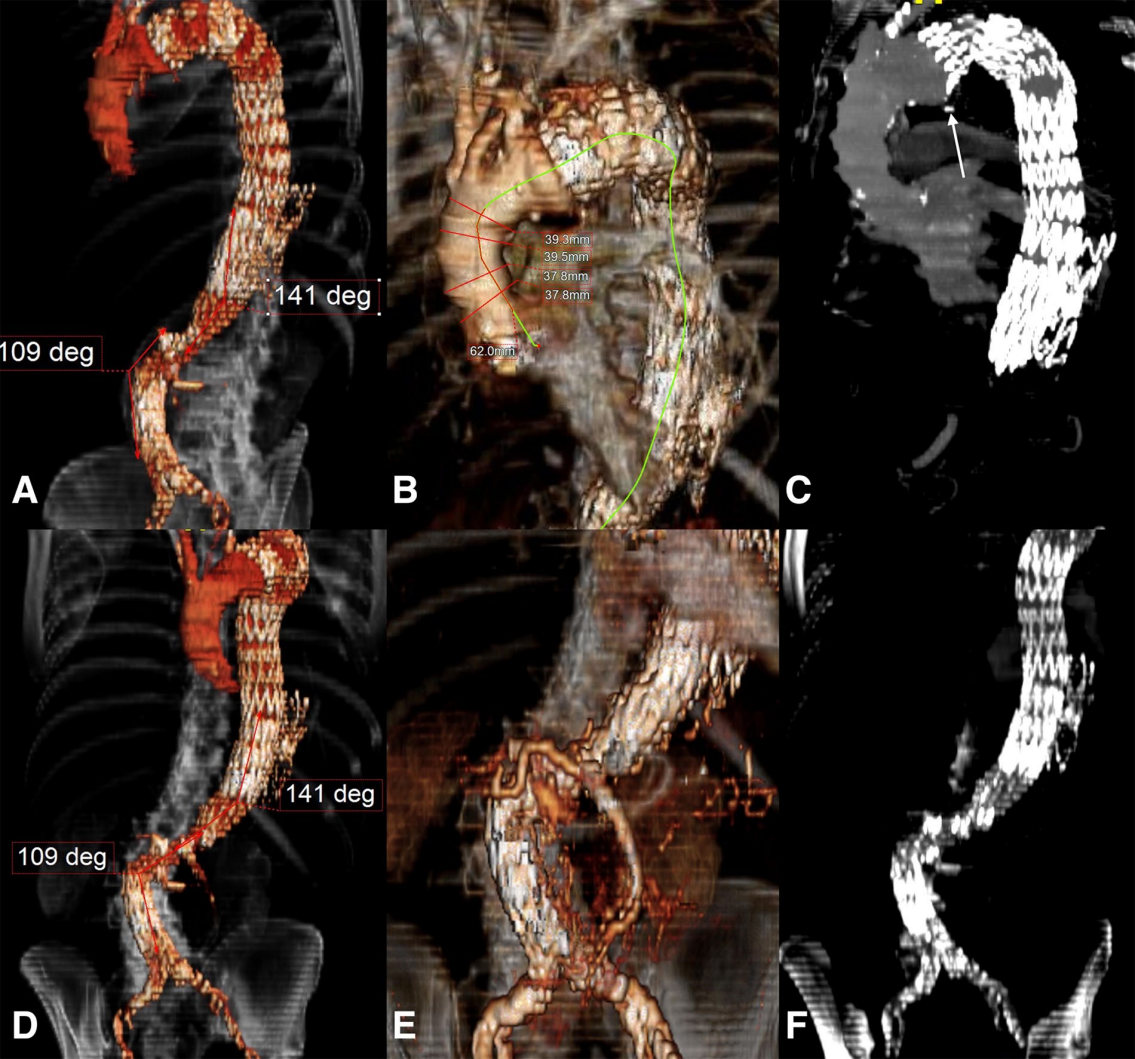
Preoperative computed tomography angiography showing (A and B) 3D and (C) multiplanar reconstruction of the migration of the previously implanted endograft, measurements (diameters and length) of the proximal sealing zone in the ascending aorta and angles of the infra-renal and supra-renal aorta are noted and the white arrow shows the retroflexion of the sealing stent of the previously implanted endograft, (D and E) 3D and (D) multiplanar reconstruction of the severe angulation (>90°) at the level of the infrarenal aorta.
